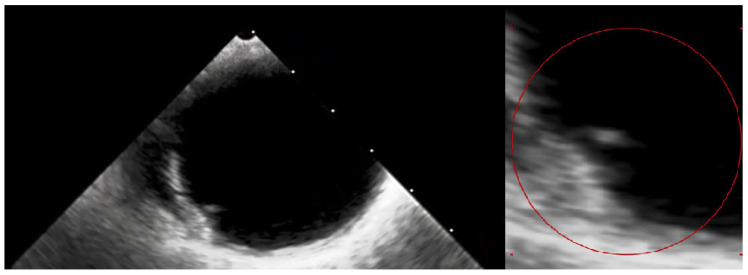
The transesophageal echocardiography failed to assess the aortic valve due to the artefacts. However, it confirmed the suspicion of the visible ruptured neointima within the aorta on the CT (Video S1—red circle). The patient again consulted with the Heart Team, and the decision about a conservative approach and administration of oral anticoagulation with vitamin K antagonists (VKAs) was sustained. At the 3-month follow-up, the patient was free from angina and syncope, and the control chest CT showed no sign of thrombus presence in the aortic region. The anatomical changes, such as the dimensions and shape of the aortic root, which occurred during TAVI, may have caused the dynamic switch in blood flow through the bioprosthetic valve, thus causing aortic remodelling. This contributes to the fact that the blood stagnates in the prosthetic sinuses, which complements Virchow’s triad and is attributed to thromboembolic events [7]. Furthermore, the ongoing structural bioprosthesis degeneration leading to fibrosis and calcification is often started by leaflet thickening and valve thrombosis [8]. The atherosclerotic plaque in the ascending aorta, or dissection of the neointima along the prosthesis, could also contribute to the thrombotic event. Aortic dissection was reported to be a rare TAVI procedure complication, occurring almost entirely as an acute condition. Regardless, there have been no previous reports of dissection of the neointima in the aortic prosthesis after ViV TAVI [9]. In our case, we suspect that a change in flow through the aorta or mechanical damage during the TAVI could have led to the dissection of the neointima and aortic thrombosis. Additionally, myocardial infarction in patients after TAVI can be the result of co-existing leaflet thrombosis and can cause difficulty in performing PCI procedures due to impaired coronary access [10]. In our case, the patient’s symptoms were most likely associated with the clinical presentation of ViV thrombosis and severe aortic graft thrombosis, in spite of the administration of SAPT—aspirin. The initial treatment with LMWH converted into VKA oral anticoagulants was sufficient to promote thrombus resolution and prevent recurrence at 3-month follow-up.
